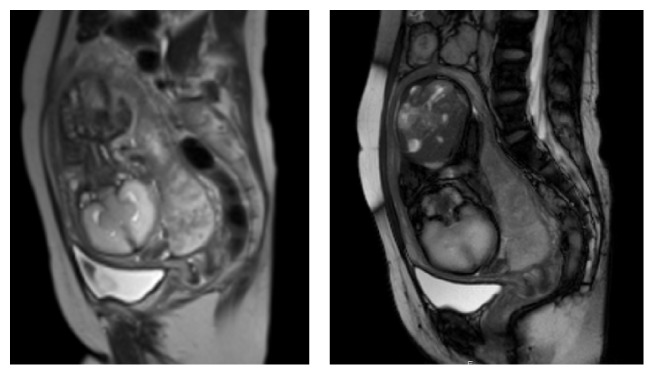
Partial placenta previa, severe oligohydramnios, and holoprosencephaly (semilobar type) by magnetic resonance imaging (MRI) at 32 weeks of gestation.
